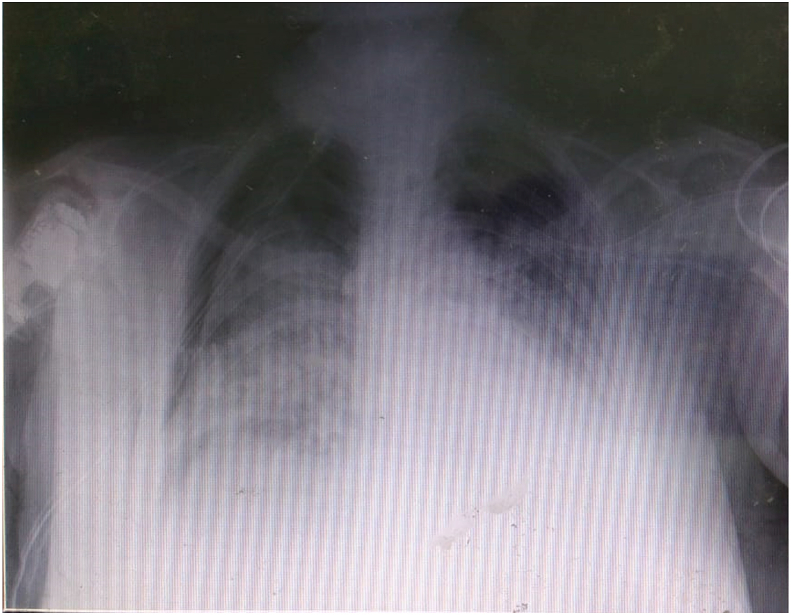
Chest -X-Ray showed a right pneumothorax.
